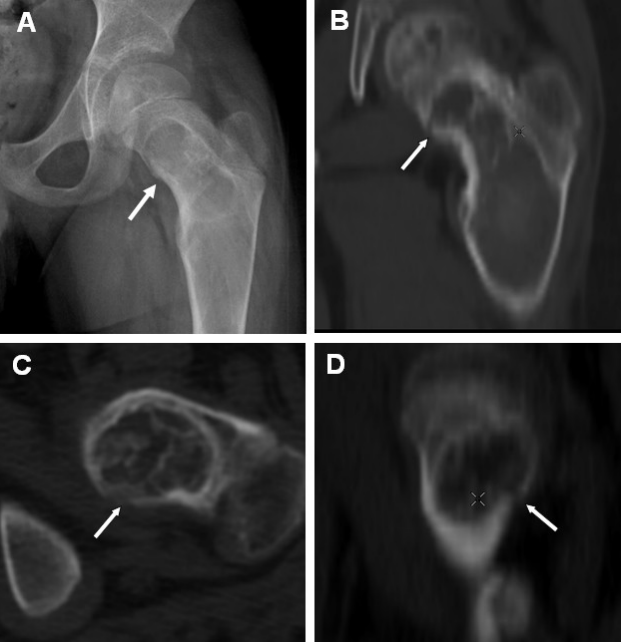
CT for detecting subtle fractures. A) This radiograph was from a 9-year-old boy who complained of new-onset, focal pain in the groin. No discernable fracture is apparent. B) Reformatted CT images of the lesion revealed a fracture in the medial proximal femur (arrows).
